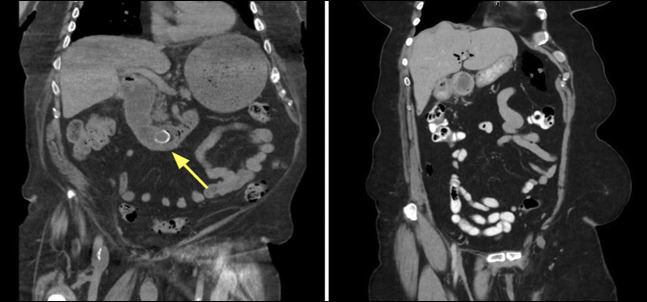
(A) Case 1: CT scan demonstrating a 35 mm gallstone in the third part of the duodenum with upstream dilatation (yellow arrow). (B) Case 2: CT scan demonstrating large ectopic gallstone, pneumobilia, and cholecystoduodenal fistula. CT, computed tomography.
